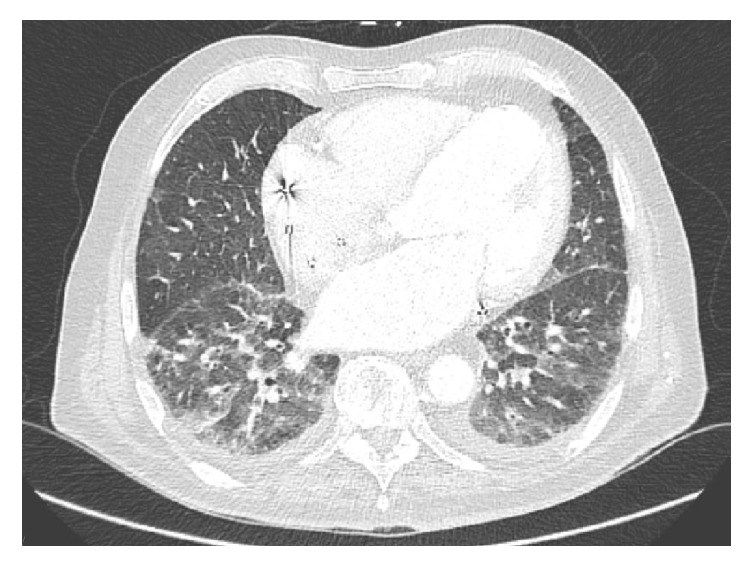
CT chest showing bilateral ground glass opacities consistent with congestive heart failure (case 2).
